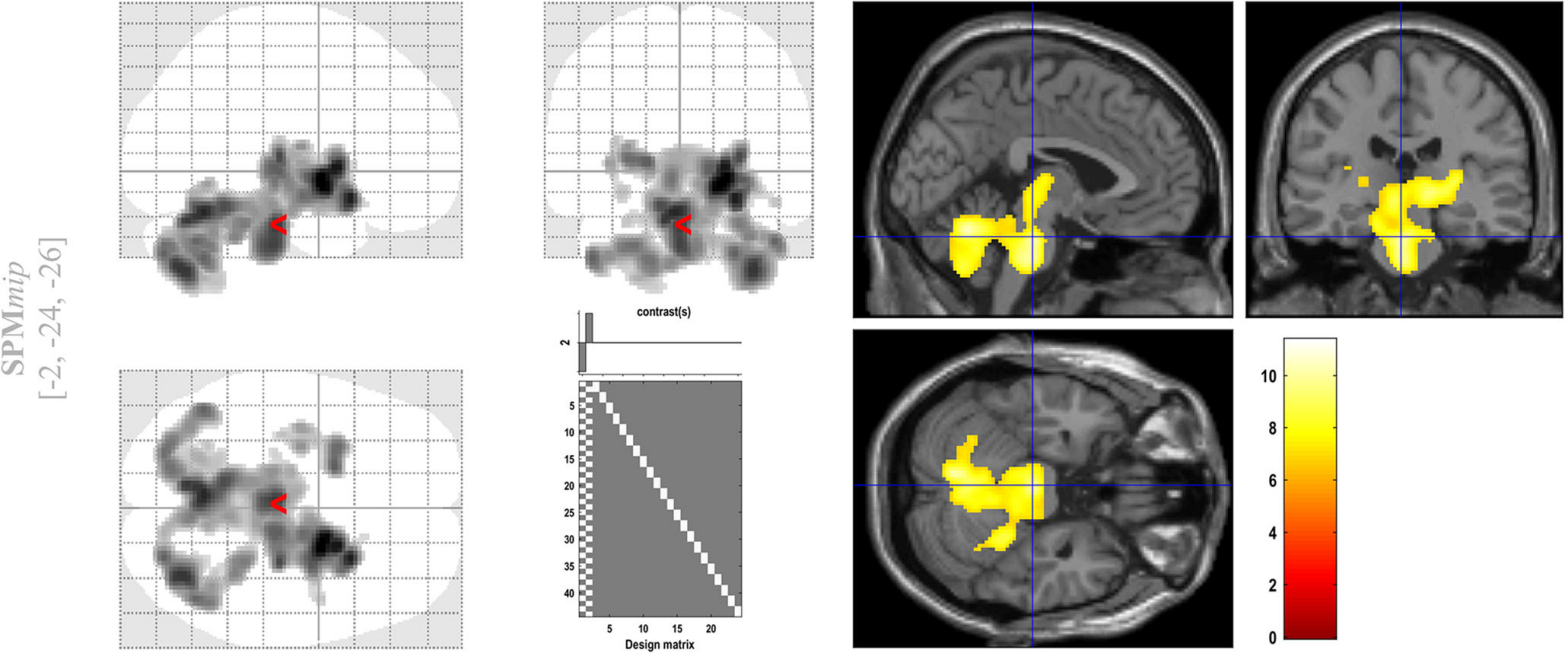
SPM results. SPM paired *t* test comparing interictal reprocessed FDG PET (rFDG PET) and interictal SPECT images showed higher activity on SPECT images with significant differences exclusively in the cerebellum, midbrain and basal ganglia. On the left, significant SPM results are shown in the form of maximum intensity projections (SPMmip) in the sagittal, coronal and axial views. On the right, *t* values obtained from the paired *t* test are superimposed on the MRI image available in SPM. Only significant *t* values are shown (colour scale from red to white)
